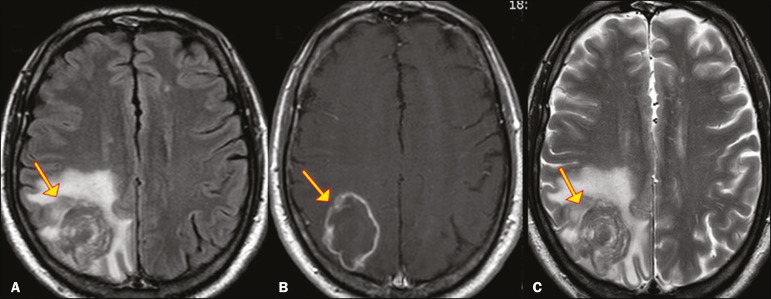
Neuroparacoccidioidomycosis. MRI sequences—FLAIR (A), contrast-enhanced axial T1-weighted (B), and axial T2-weighted (C)—identifying an expansile lesion in the right parietal lobe. On the FLAIR and T2-weighted images, the lesion showed a hypointense signal with peripheral enhancement and vasogenic edema surrounding the lesion, as well as having an onion-skin appearance on the T2-weighted images.
